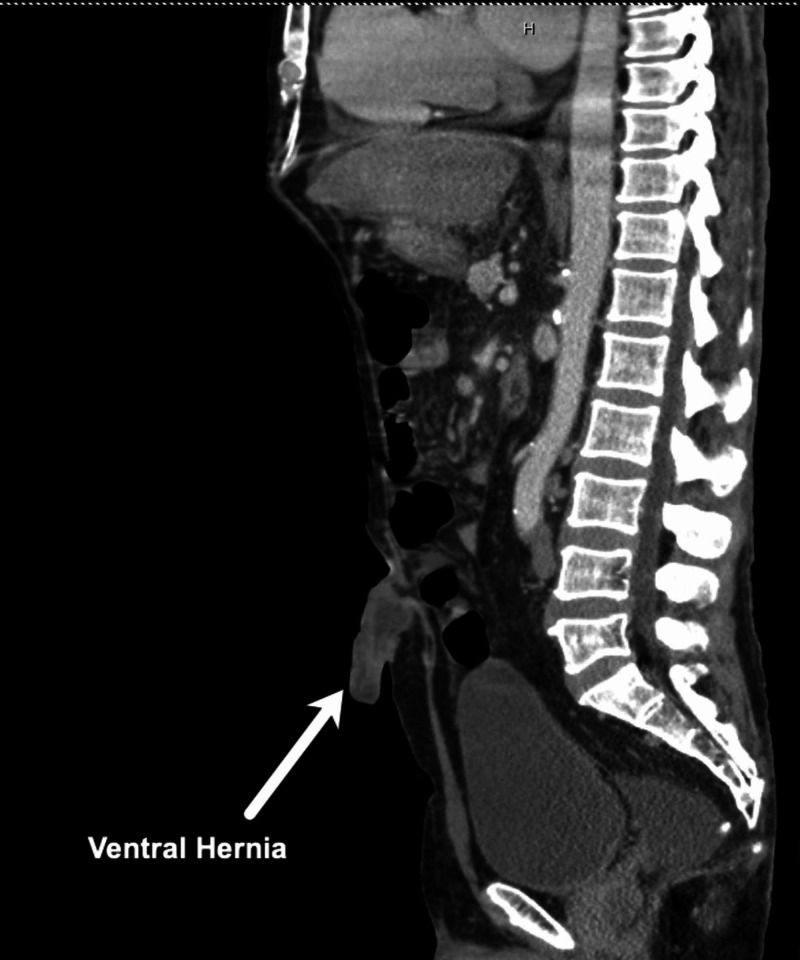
Case 1: Sagittal CT scan.
